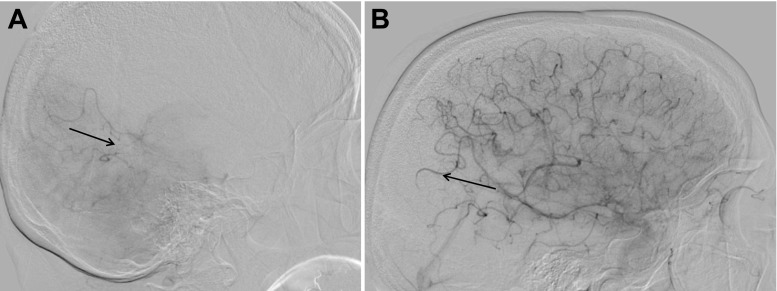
The DSA of the dominant vertebral artery in this 79-year-old woman with occlusion of the internal carotid artery shows the retrograde filling of the posterior parietal branches of the MCA (a). Due to the still active collaterals from the vertebrobasilar system, not visible in the selective DSA, the interventional team rated this as TICI 2b based on the stagnating flow and diminished temporooccipital blush (b). Quality control MR the next day found neither EE nor DWI lesions in this area
